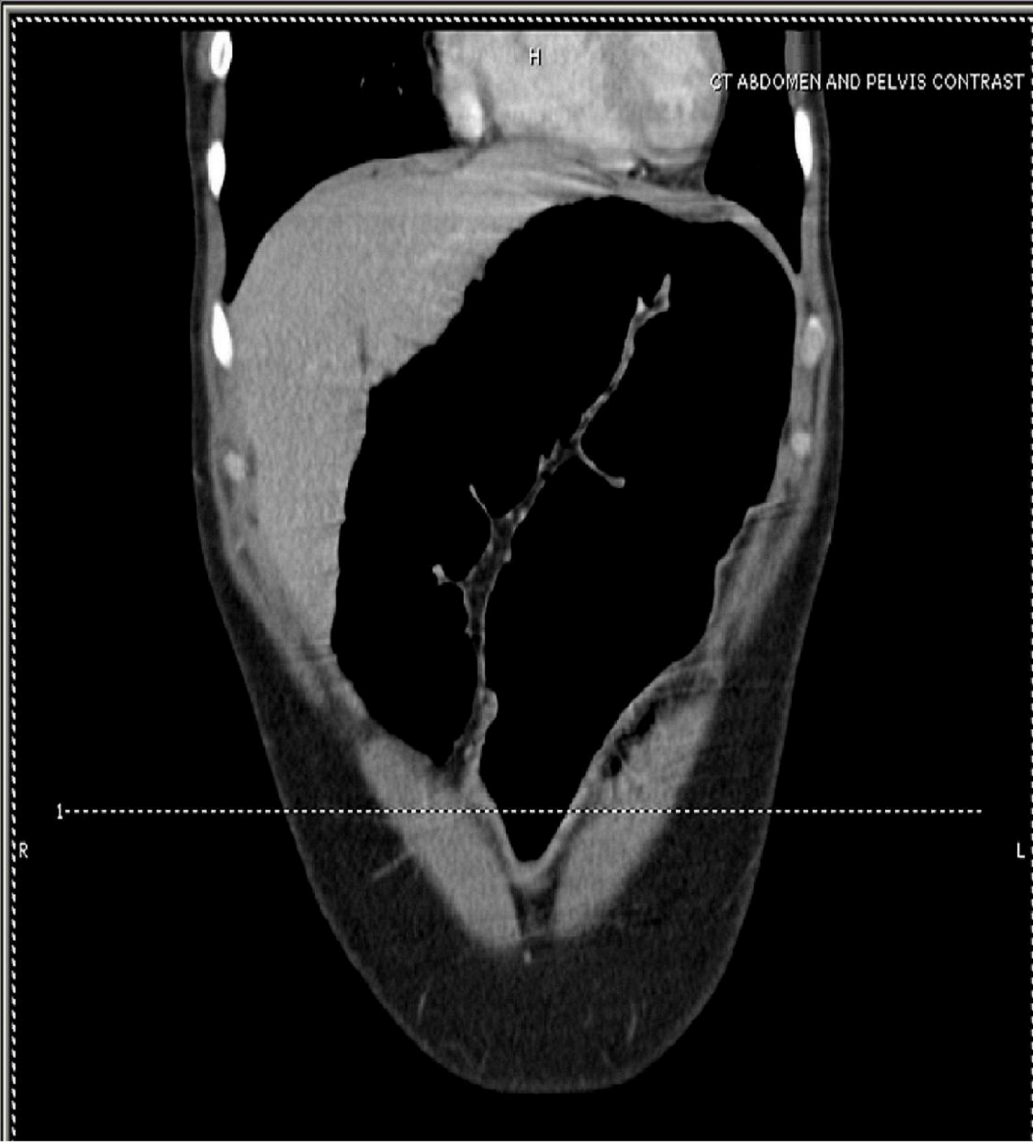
CT of the Abdomen Coronal image of the contrast-enhanced CT of the abdomen showing a significantly distended sigmoid colon looped in an inverted "U" without haustral markings.
